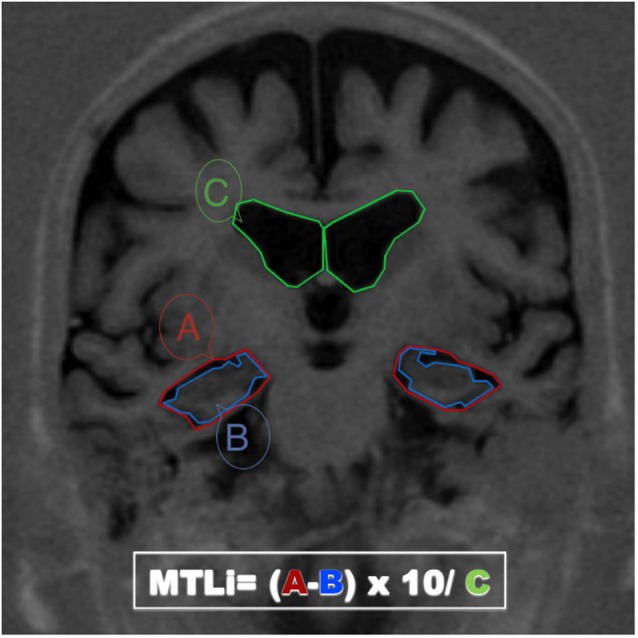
MRI coronal section passing through the interpeduncular fosae. The boundaries of the three areas needed for calculating the Medial Temporal Atrophy index (MTAi) and derived methods have been drawn in three different colors: (1) the medial temporal lobe region (A, red), defined in a coronal brain slide as the space bordered in its inferior side by the tentorium cerebelli, in its medial side by the cerebral peduncles, in its upper side by the roof of the temporal horn of the lateral ventricle and in its lateral side by the colateral sulcus and a straight-line linking the colateral sulcus with the lateral edge of the temporal horn of the lateral ventricle; (2) the parenchima within the medial temporal region, that includes the hippocampus and the parahippocampal girus (B, blue); and (3) the body of the ipsilateral lateral ventricle (C, green).
